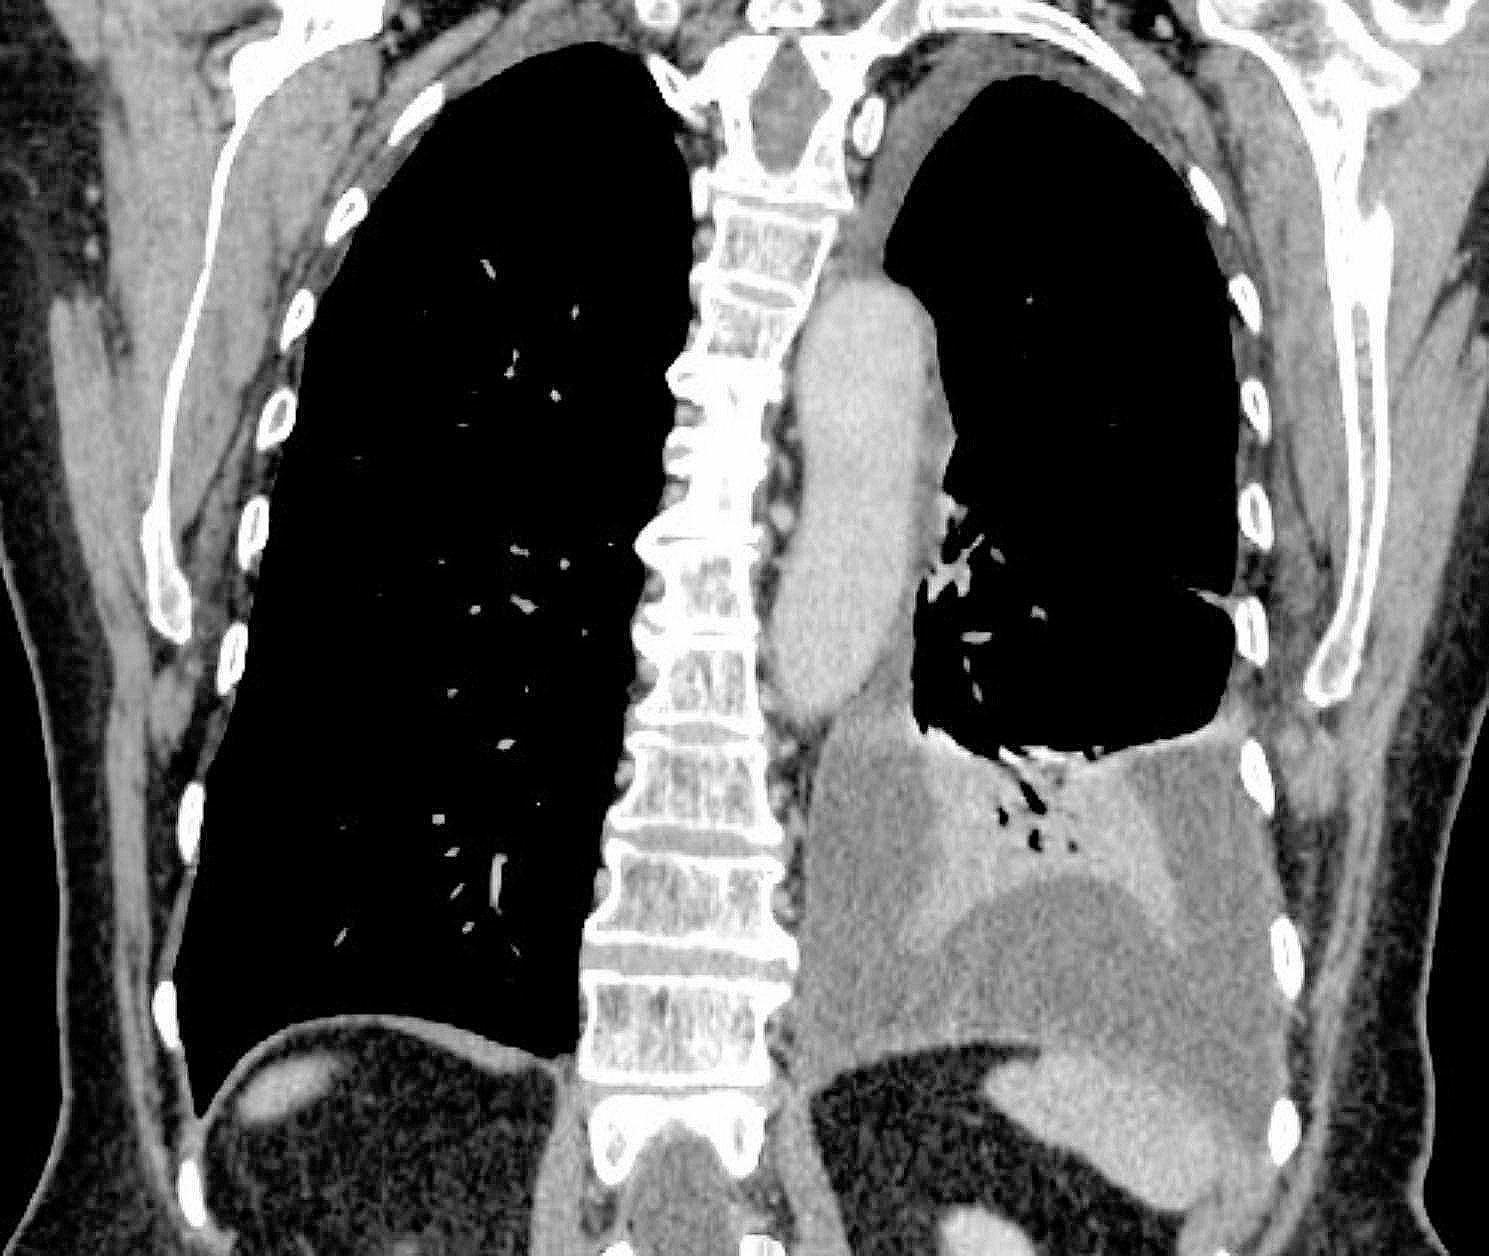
CT scan of pleural fluid
